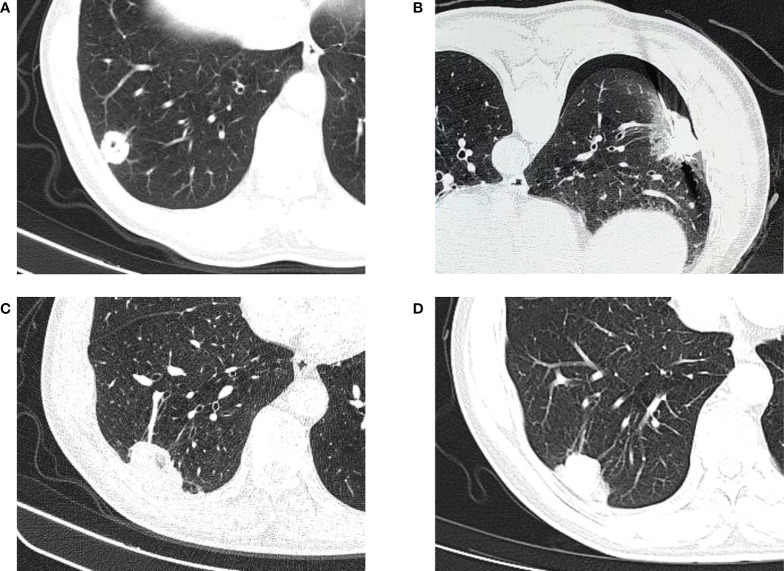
CT-guided percutaneous RFA. (A) CT scan performed before RFA, showing a lesion in the lower right lobe. (B) CT−guided percutaneous RFA of the lesion. (C) CT scan performed 1 month after the procedure. (D) CT scan performed 4 months after the procedure.
